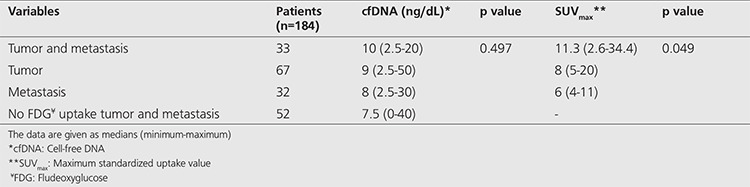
Comparison of tumor and metastasis presence with cell-free DNA and SUV_max_ values in oncologic patients
